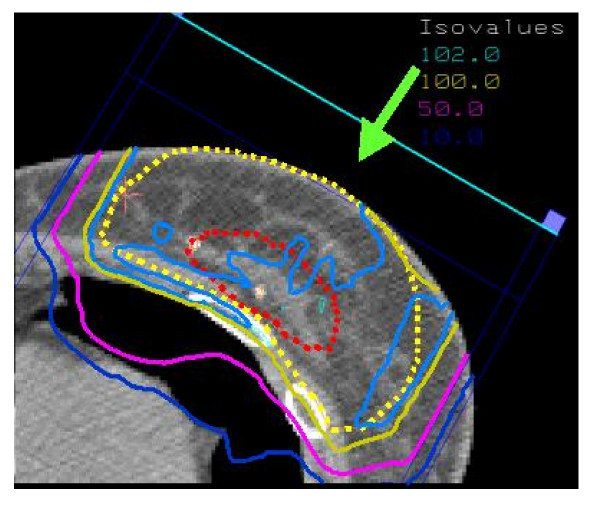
Dose distribution (protons) in an axial CT slice through the center of the breast for an early breast cancer patient treated with partial breast irradiation. The isodose contours are represented by different colors (corresponding values are displayed on the upper-right border of the figure).
